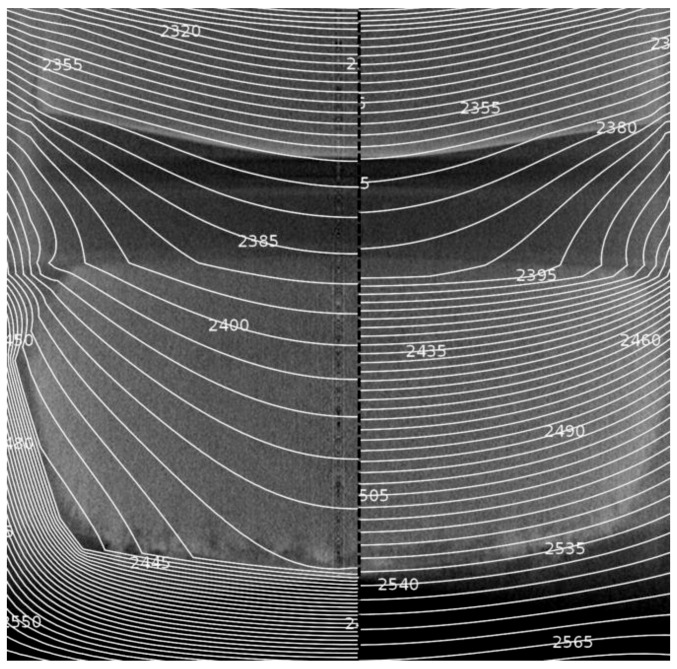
In situ CT data for the crucible at a growth time of 115 h with an overlay indicating the results from the numerical modeling of the temperature field. On the left half, the discussed thermal properties of the graphite skeleton and the anisotropic needle-like structure are taken into account for the source area. In comparison, the right side shows the temperature field if the initial thermal conductivity of the SiC powder is assumed in the whole source area.
